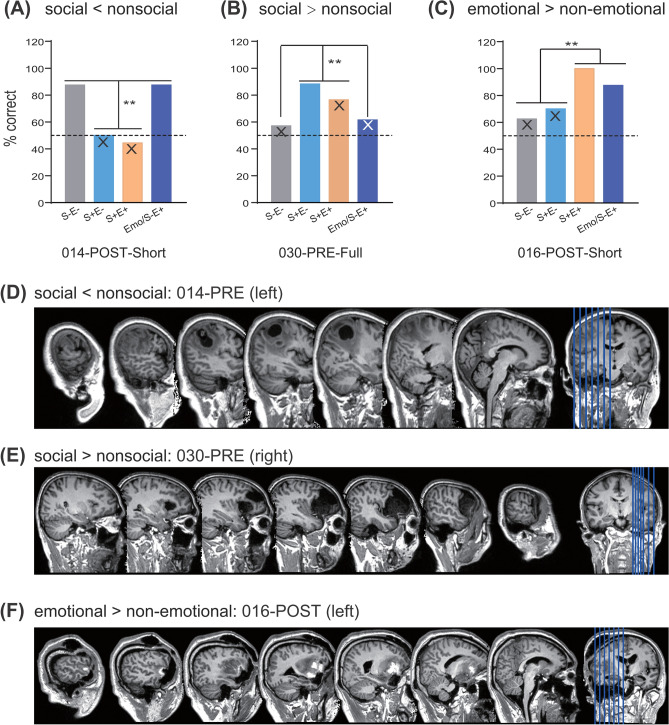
Behavioral performances (A–C) and lesions (D–F) of cases showing significant dissociations that survived all the validation analyses. Asterisks indicate significant dissociations, when compared with 28 healthy controls and controlling for three demographic variables (, *p* < .01, single-case dissociation tests^38^). The X marks indicate significant deficits in a given category compared with 28 healthy controls while controlling for three demographic variables (one-tailed *p* < .05,^38^). The brain slices are visualized using MRIcron^69^. Note that D shows the presurgical MRI scan of Patient 014, who did not have available postsurgical MRI scans. S−E−, nonsocial non-emotional words; S+E−, social non-emotional words; S+E+, social emotional words; Emo/S−E+, emotional state words with relatively low social ratings. *PRE* examined before surgery, *POST* examined shortly after surgery, *Full* the full-version test, *Short* the short-version test.
